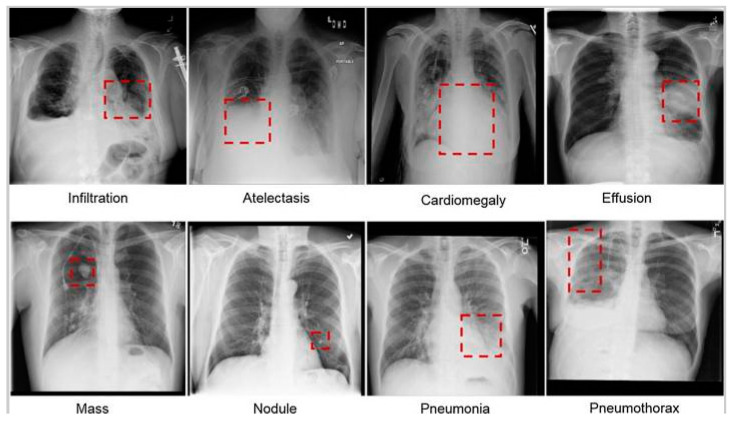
The different types of lung infections. Eight of the most common lung diseases; namely, infiltration, atelectasis, cardiac hypertrophy, effusion, lumps, nodules, pneumonia, and pneumothorax; observed in the chest radiographs [12].
